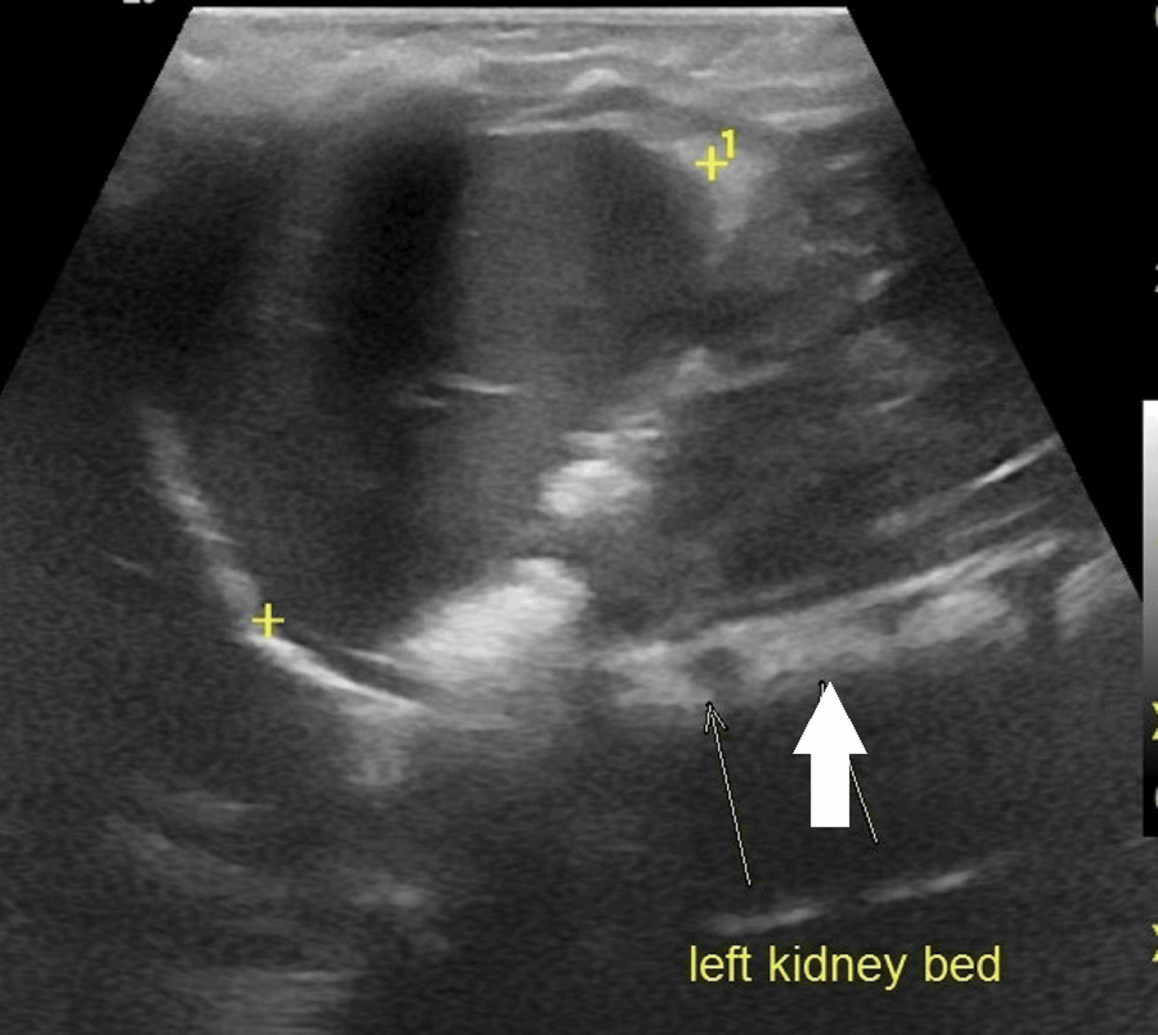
Follow-up post-operative ultrasound showing an empty left kidney bed.
